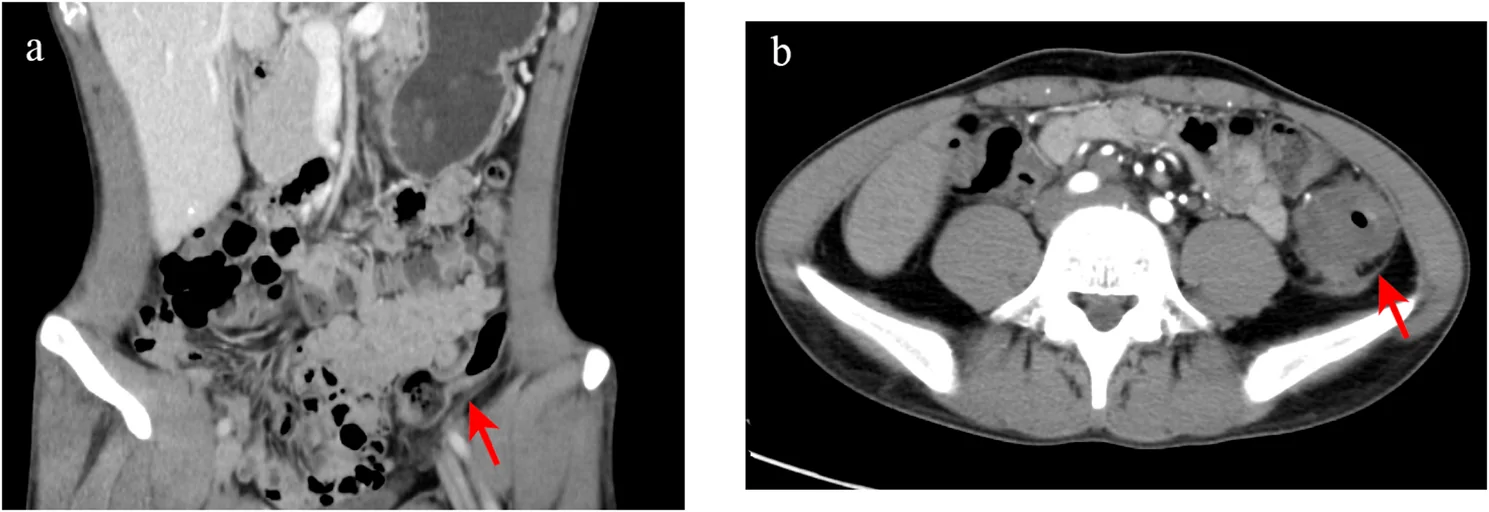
Abdominal computed tomography (CT): a Coronal view demonstrates stenosis of the sigmoid colon lumen. b Axial view shows marked wall thickening accompanied by several mildly enlarged regional lymph nodes
